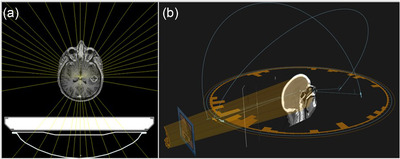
Representative beam arrangements for (a) MRI‐guided radiotherapy (MRgRT) co‐planar fixed‐field IMRT and (b) noncoplanar volumetrically modulated arc therapy (VMAT) on a conventional c‐arm linear accelerator
